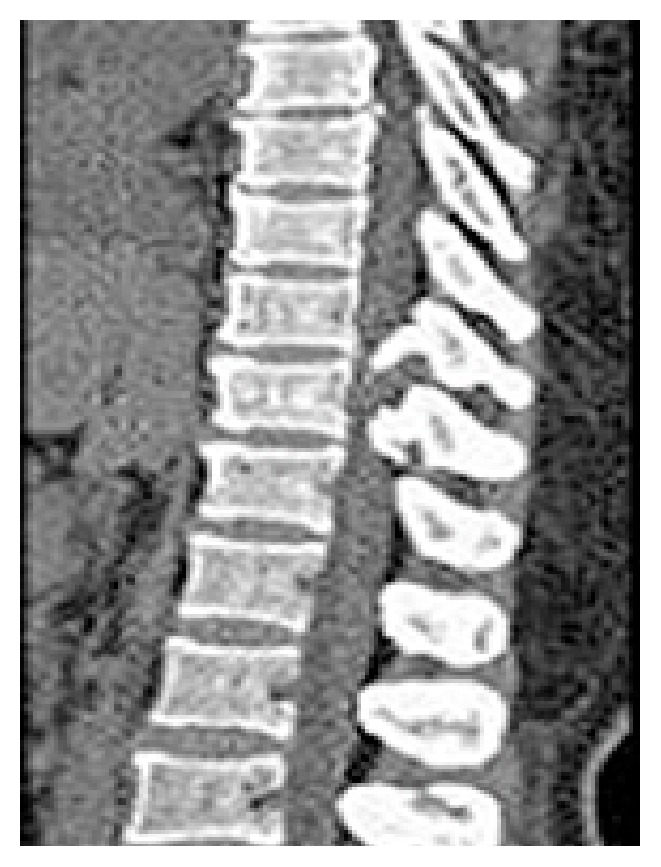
CT thoracic spine shows T10-T11 vertebral body and disc regeneration.
